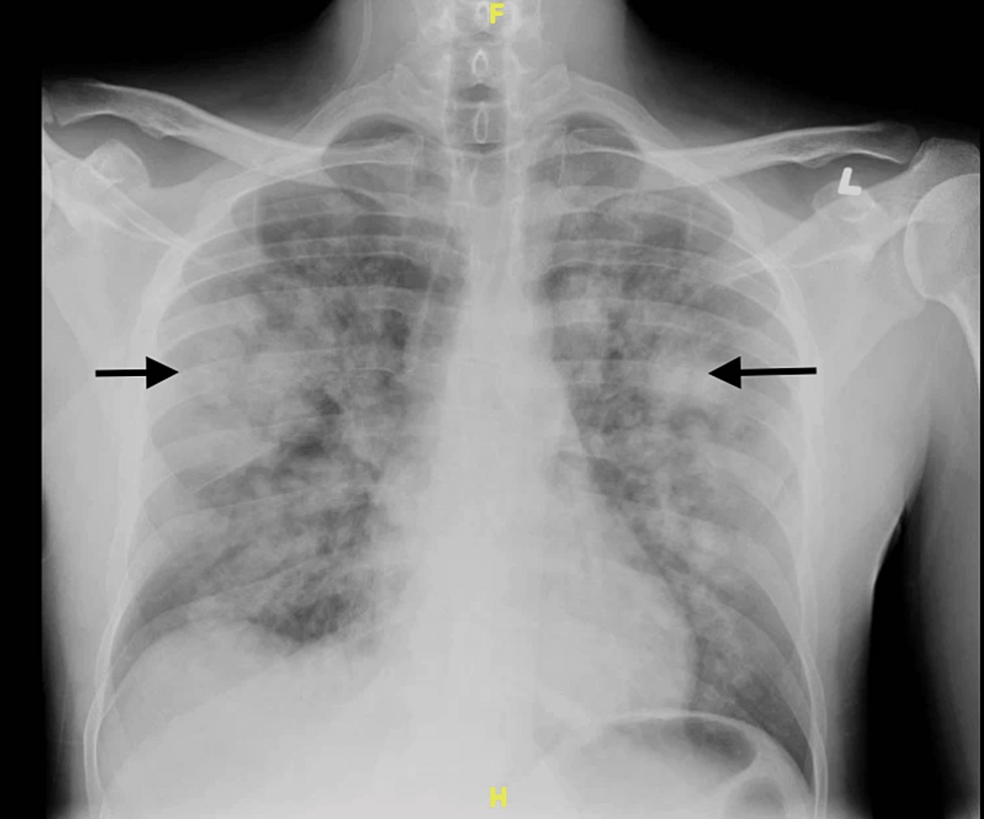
X-ray chest AP view of the chest showing areas of consolidation. Black arrows showing areas of consolidation.
